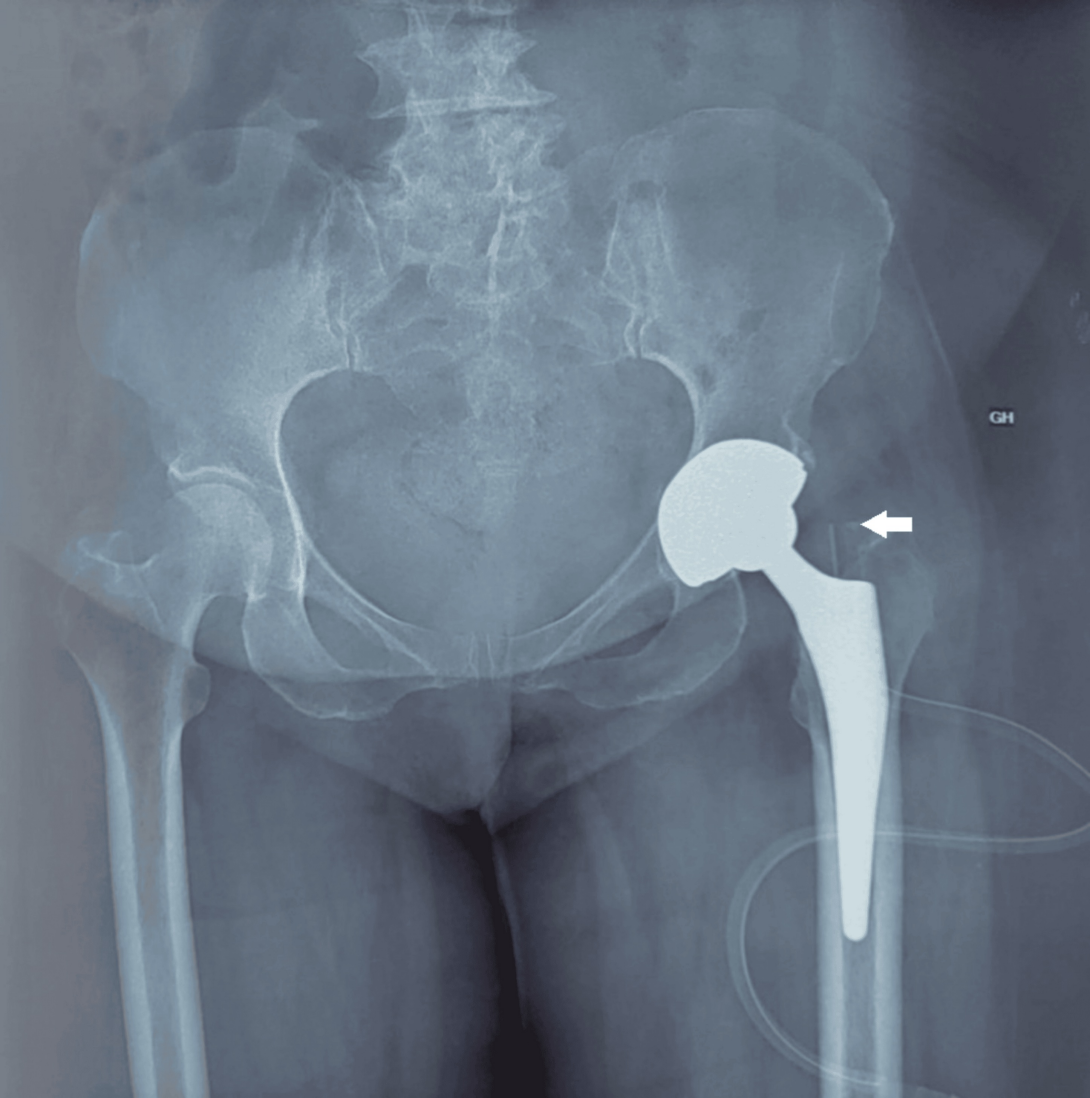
Postoperative standard frontal X-ray of the pelvis
